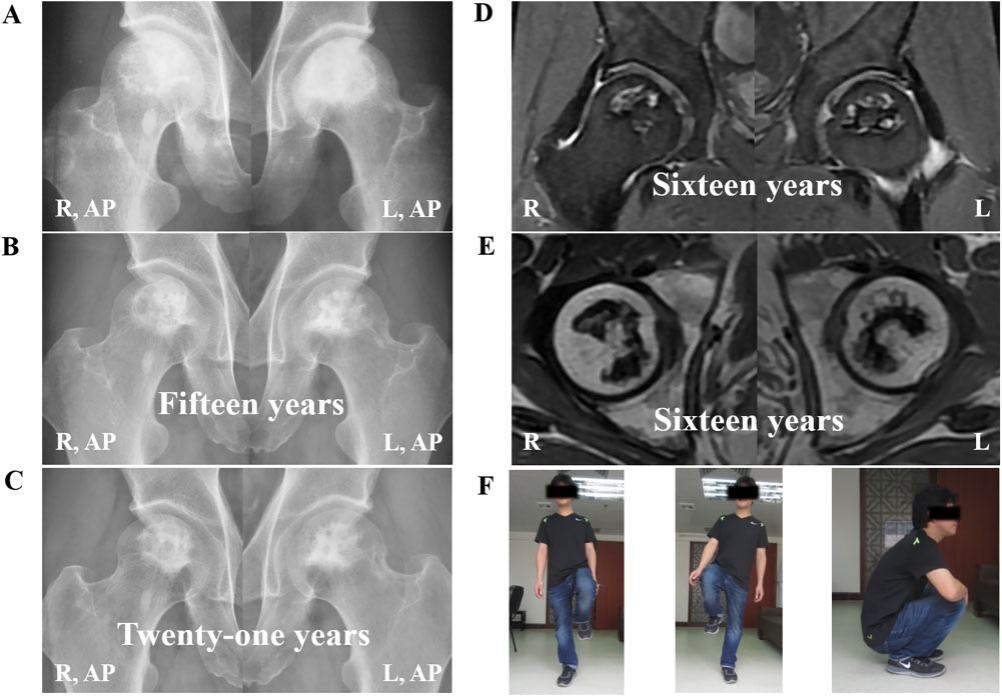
A 48-year-old man (Patient 25) who has idiopathic necrosis of the femoral head. (A) Frog-leg lateral radiographs and anteroposterior radiographs show bilateral hips with stage II disease and type A at his initial diagnosis. (B) Without special treatment for 15 years, the patient was free from symptoms and had no collapse of the femoral heads bilaterally on frog-leg lateral radiographs and anteroposterior radiographs. (C) After 21 years, the patient was free from symptoms and had no radiographic progression of the bilateral femoral head. (D and E) MRI image of the suffered hip obtained 16 years later. (F) At present, the patient was free from symptoms and had good function.
